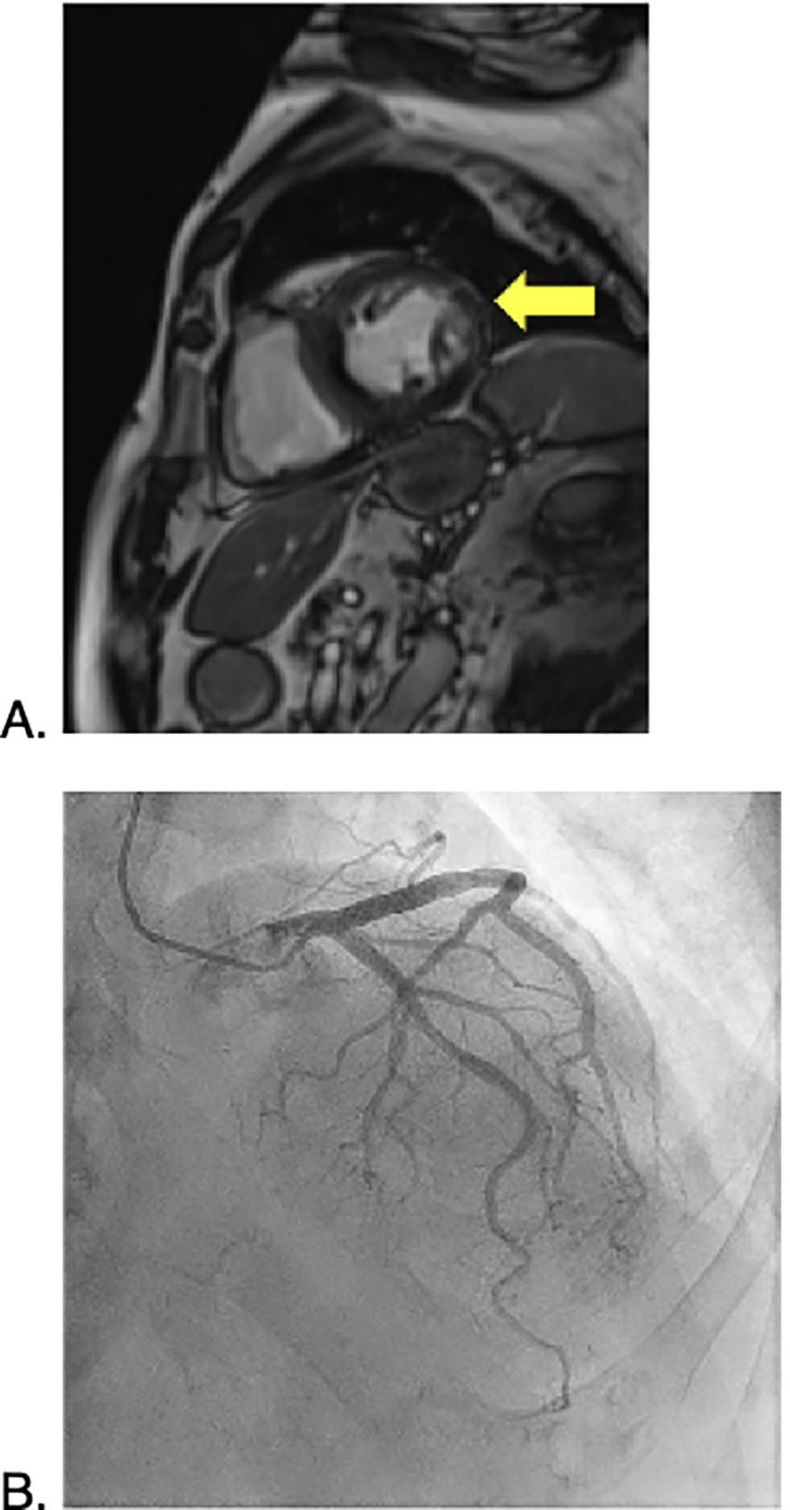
Cardiac MRI (A) showing mild edema signal in the left ventricular mid lateral wall with early and delayed moderate enhancement consistent with myocarditis, and coronary angiography (B) showing mild atherosclerosis.
